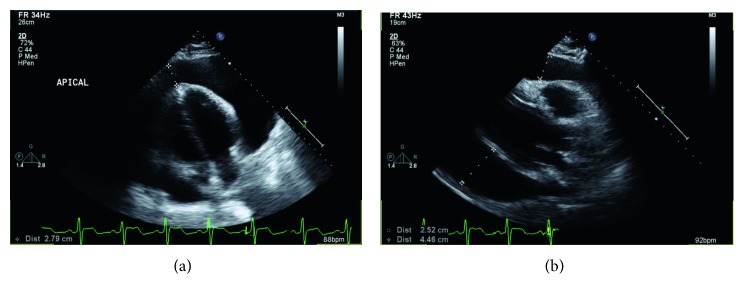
Apical (a) and parasternal (b) long axis views of the hemorrhagic pericardial effusion on presentation.
